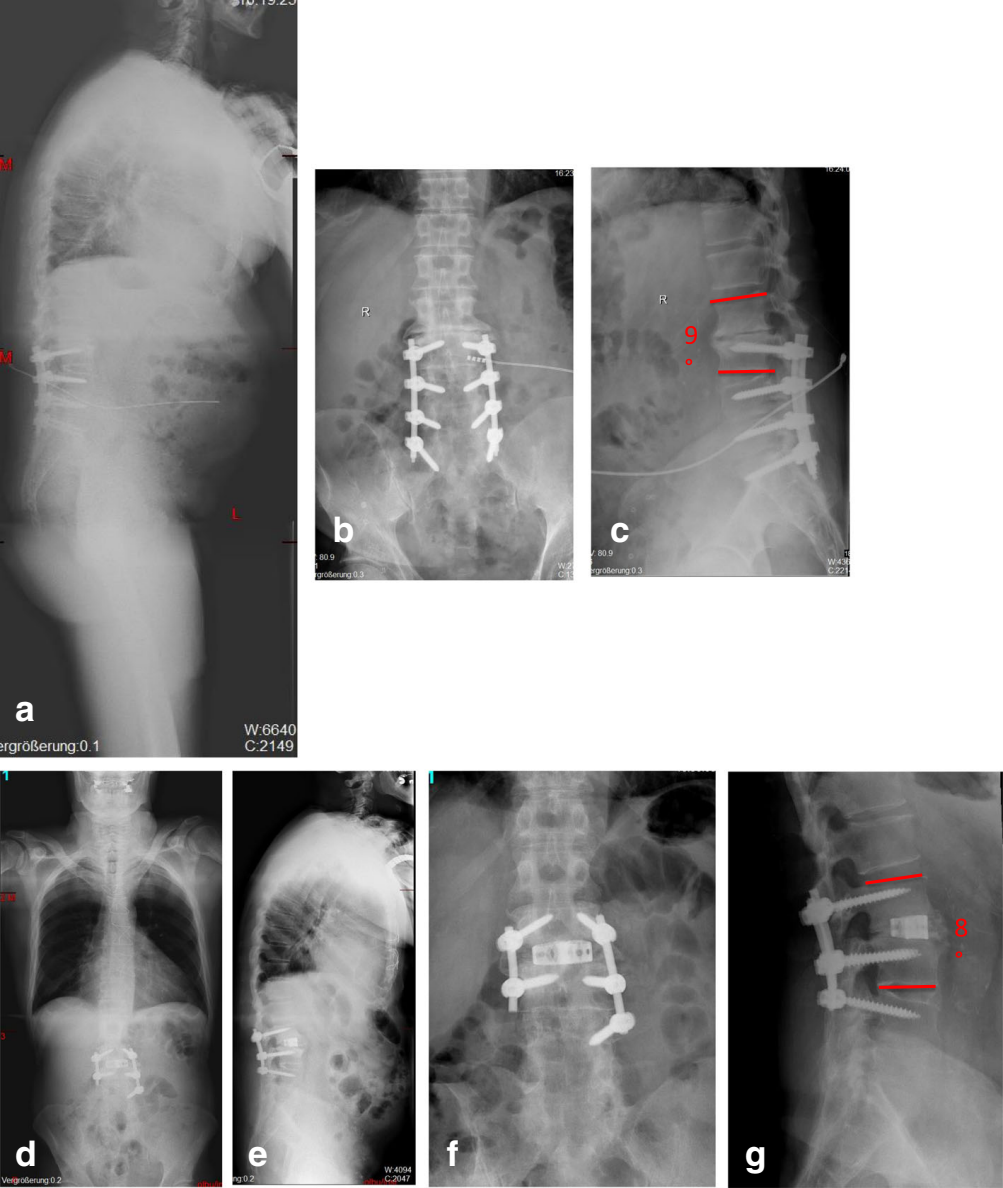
65- year old male with fixed adjacent segment disease L2/3 post posterior instrumentation and fusion L3-S1 including the implantation of an neurological stimulator in an outside hopsital. Figure a shows the sagittal whole-spine x-ray, Figure b the standing ap x-ray of the lumbar spine, Figure c the lateral x-ray including the measurements of the deformity. Figures d and e display the postop standing whole-spine x-rays, Figures f and g the lumbar x-rays including the measurements
